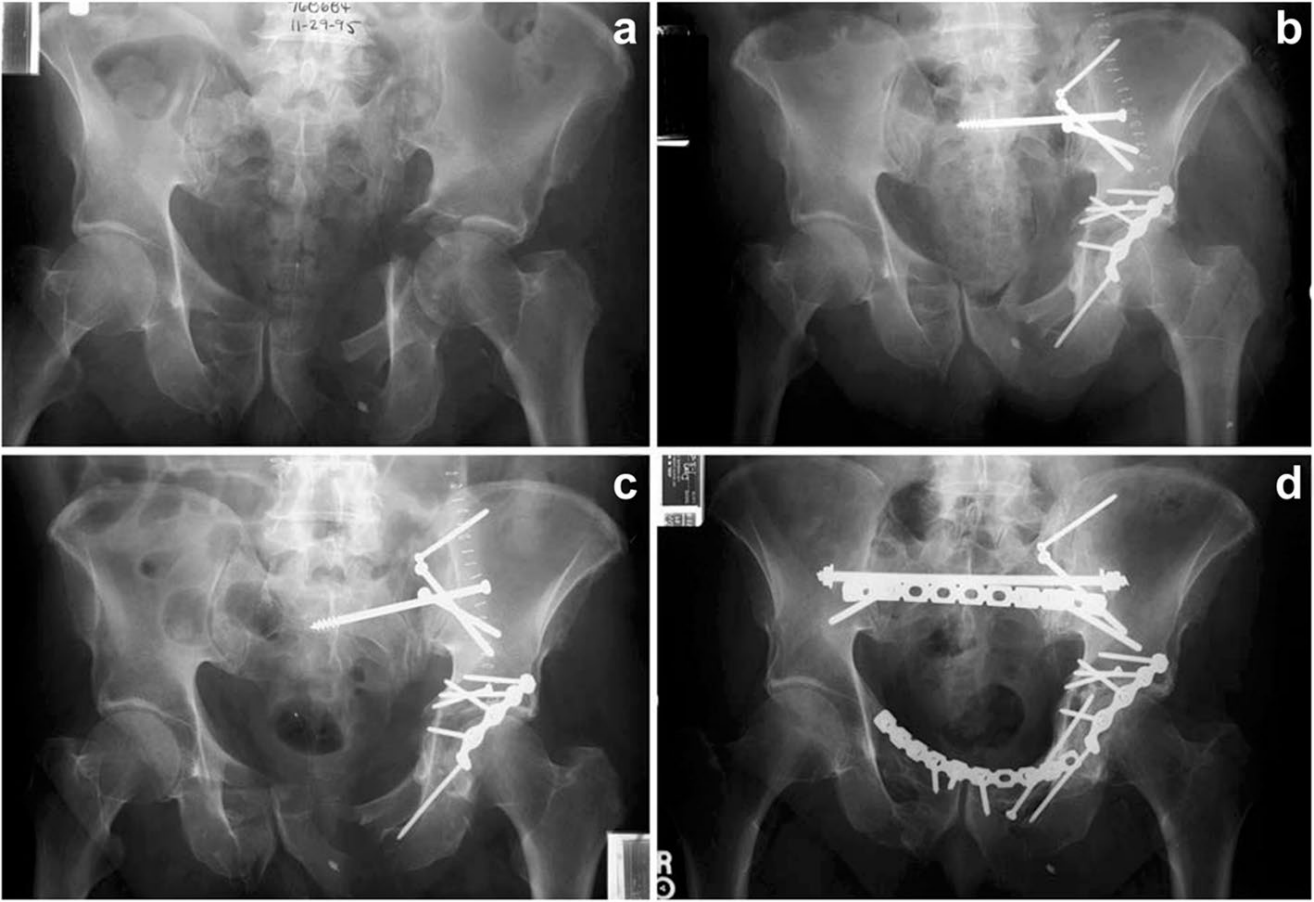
Radiographic images of a combined left SI fracture-dislocation and T-shaped acetabular fracture (A). After fracture fixation (B), the posterior pelvic fixation failed (C) necessitating revision with a trans-sacral screw, posterior tension band plate, and anterior ring fixation (D)
